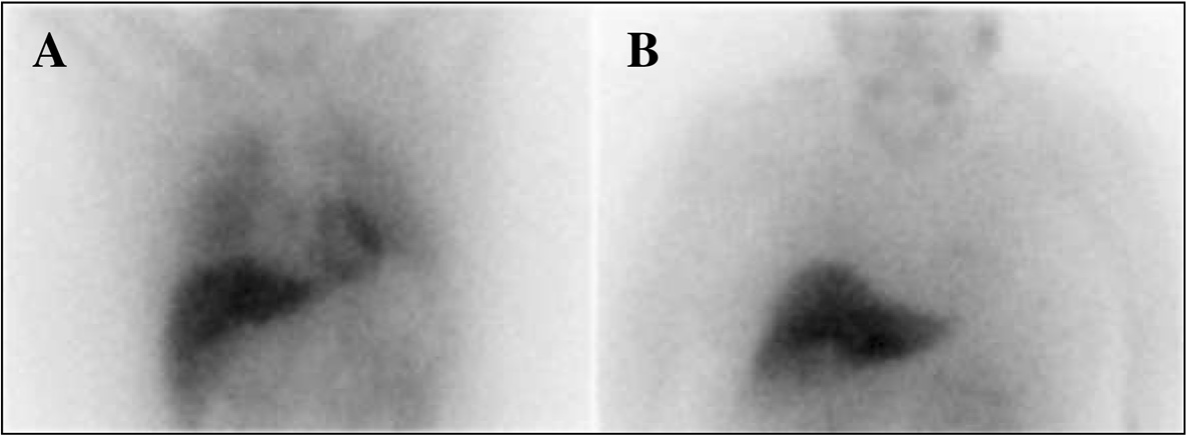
Planar 123-I MIBG cardiac scintigraphy images. a. ^123^MIBG cardiac scintigraphy from a patient with multiple system atrophy with normal cardiac MIBG uptake in delayed image. b. ^123^MIBG cardiac scintigraphy from a patient with Parkinson’s disease with marked reduction of cardiac MIBG uptake in delayed image
